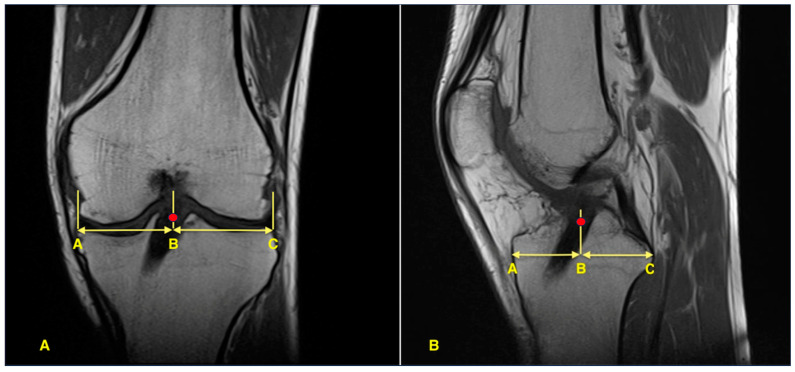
Measurement of tibial tunnel position on post-operative MRI. The red dot indicates the center of the graft. (A) Coronal view showing mediolateral tunnel position calculated as the percentage ratio AB/AC. (B) Sagittal view showing anteroposterior tunnel location calculated as the percentage ratio AB/AC.
